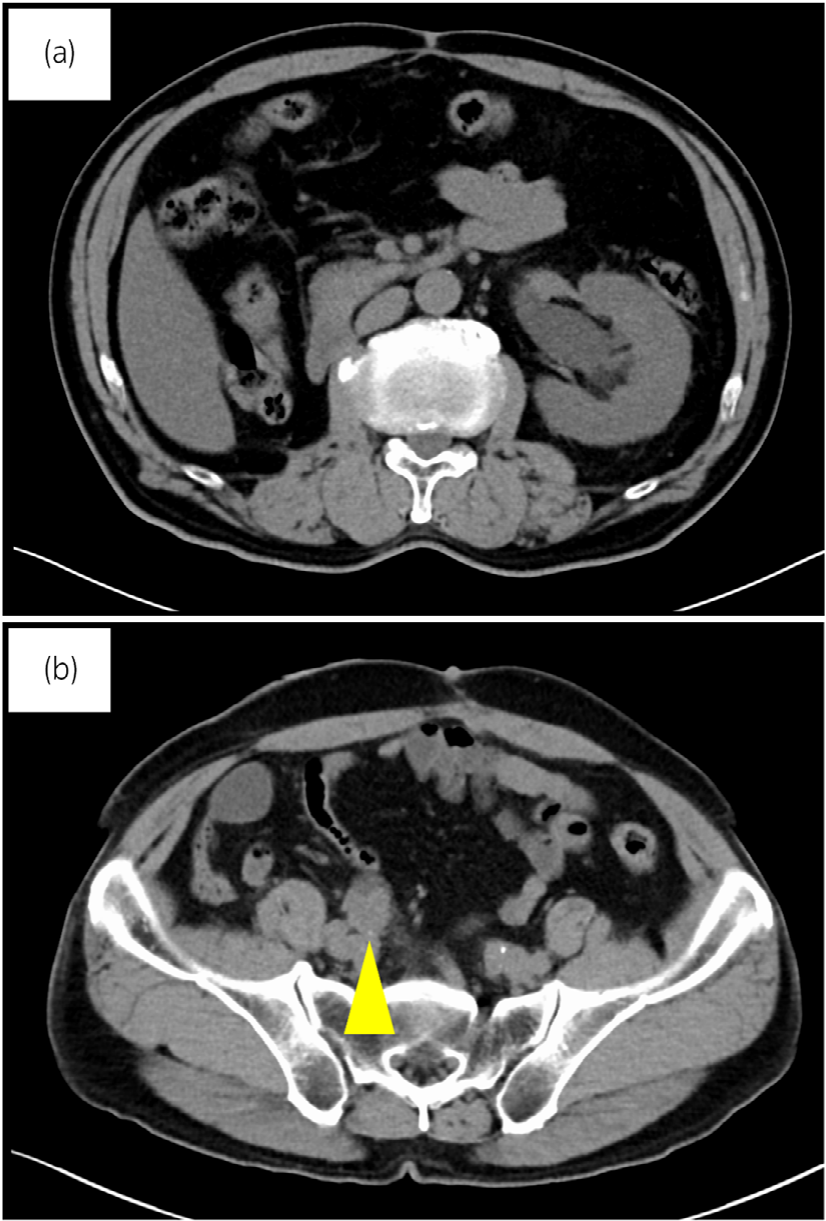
Axial CT showing (a) left hydronephrosis and acute pyelonephritis and (b) a recurrent mass at the left uretero‐ileal anastomosis (yellow arrowhead).
